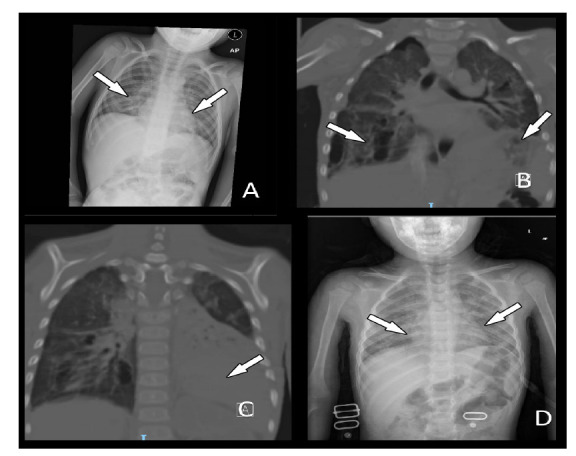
Progression of chest radiography (CXR) and computed tomography (CT) findings in a pediatric COVID-19 patient. A) CXR of the patient on admission showed bilateral mid-lung zone opacifications. B&C) During admission to the pediatric intensive care unit, coronal CT images of the same patient showed multiple right sided pneumatoceles, bilateral ground-glass opacification, and left lower lobe consolidation. D) CXR of the patient at the 3-month post-discharge follow-up showed recovering bilateral ground-glass opacifications.
